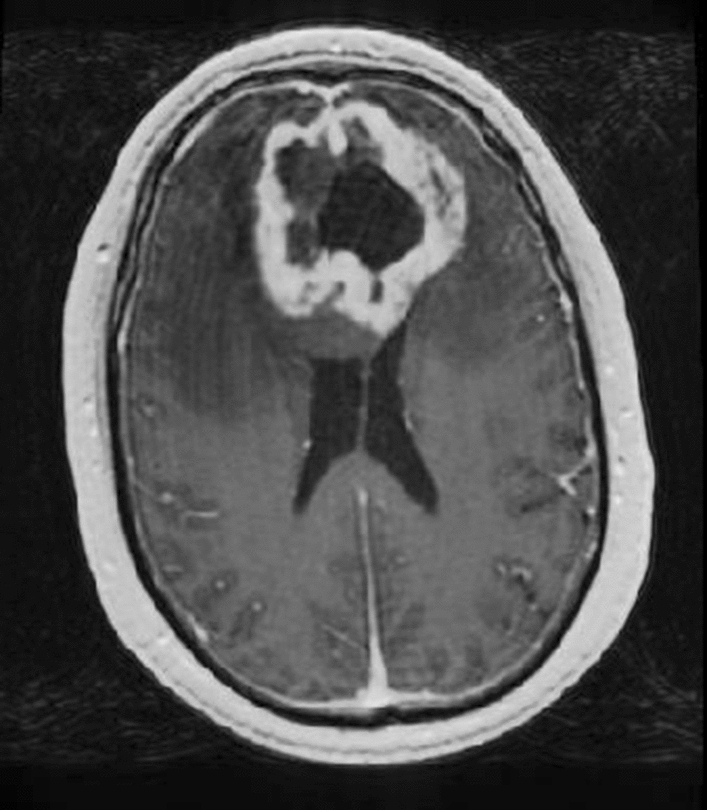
Post-gadolinium-based contrast-enhanced T1-weighted axial image shows a ring enhancing lesion, in this case a glioblastoma. The peripheral enhancement is caused by accumulation of the contrast agent due to disruption of the BBB
